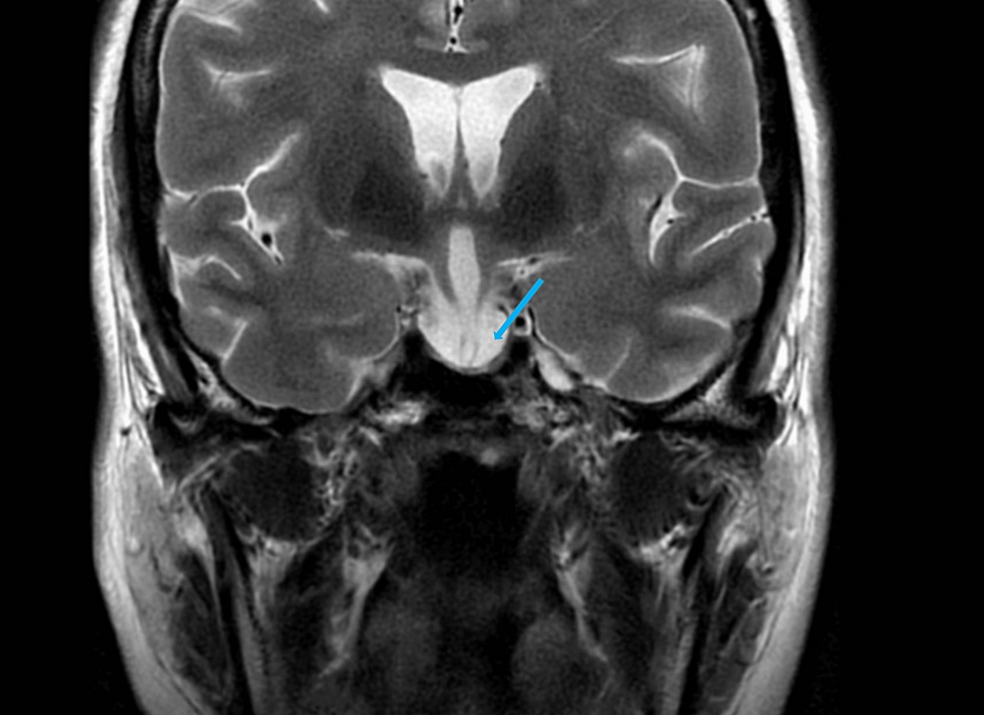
Coronal T2-weighted imaging showed the sella turcica filled with cerebrospinal fluid (blue arrow).
